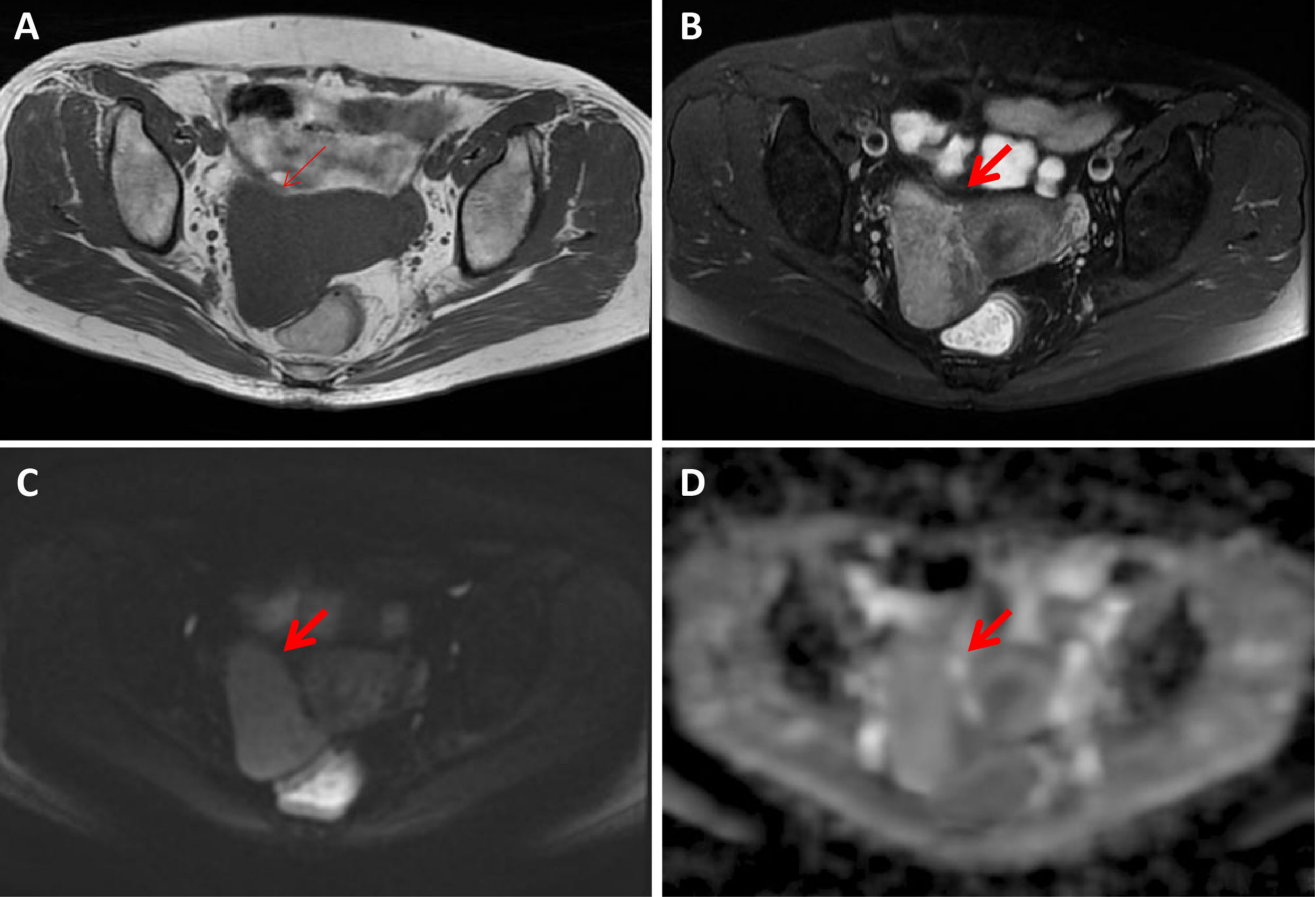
A 56-year-old woman with a hydropic degeneration leiomyoma. The mass was detected in the uterine right wall (arrow) with isointensity on T1WI (A) and heterogenous iso-to-hyperintensity on T2WI images (B). The tumor showed high signal intensity on diffusion-weighted MR image (C) with a high ADC value (ADC = 1339 × 10^-6^ mm^2^/sec) (D).
